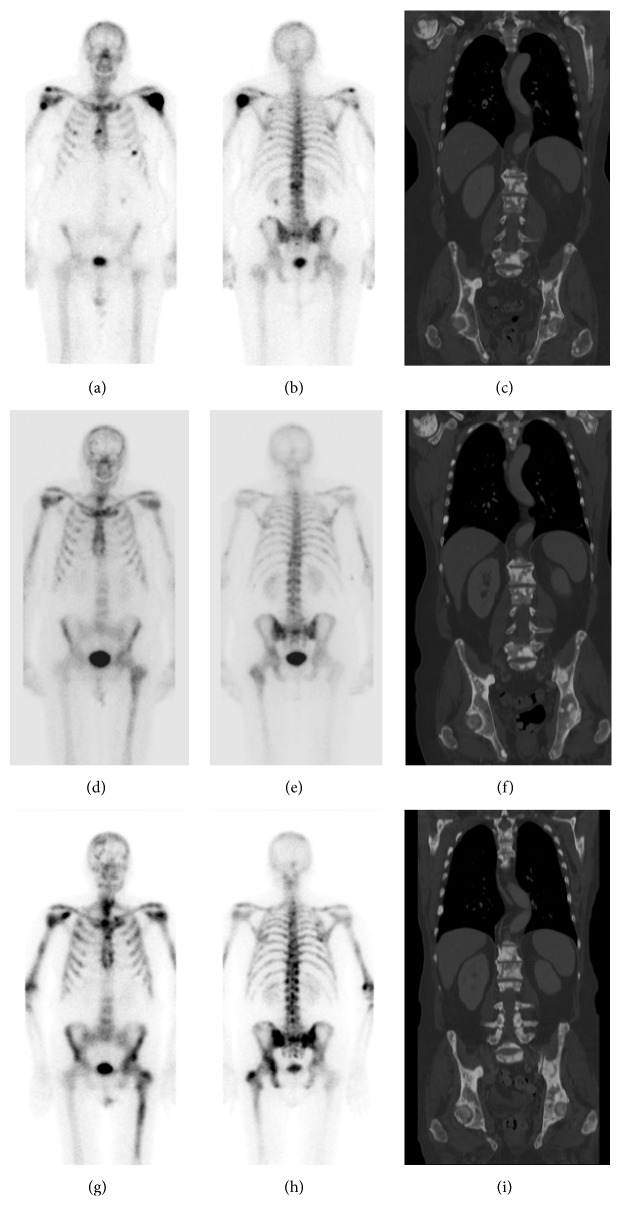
Case #2 of a 65-year-old man. Anterior and posterior bone scan, and CT at baseline (a, b, c), within 3 months after completion of Radium-223 (d, e, f), and 6–12 months after Radium-223 (g, h, i). Imaging findings are summarized in Table 2.
